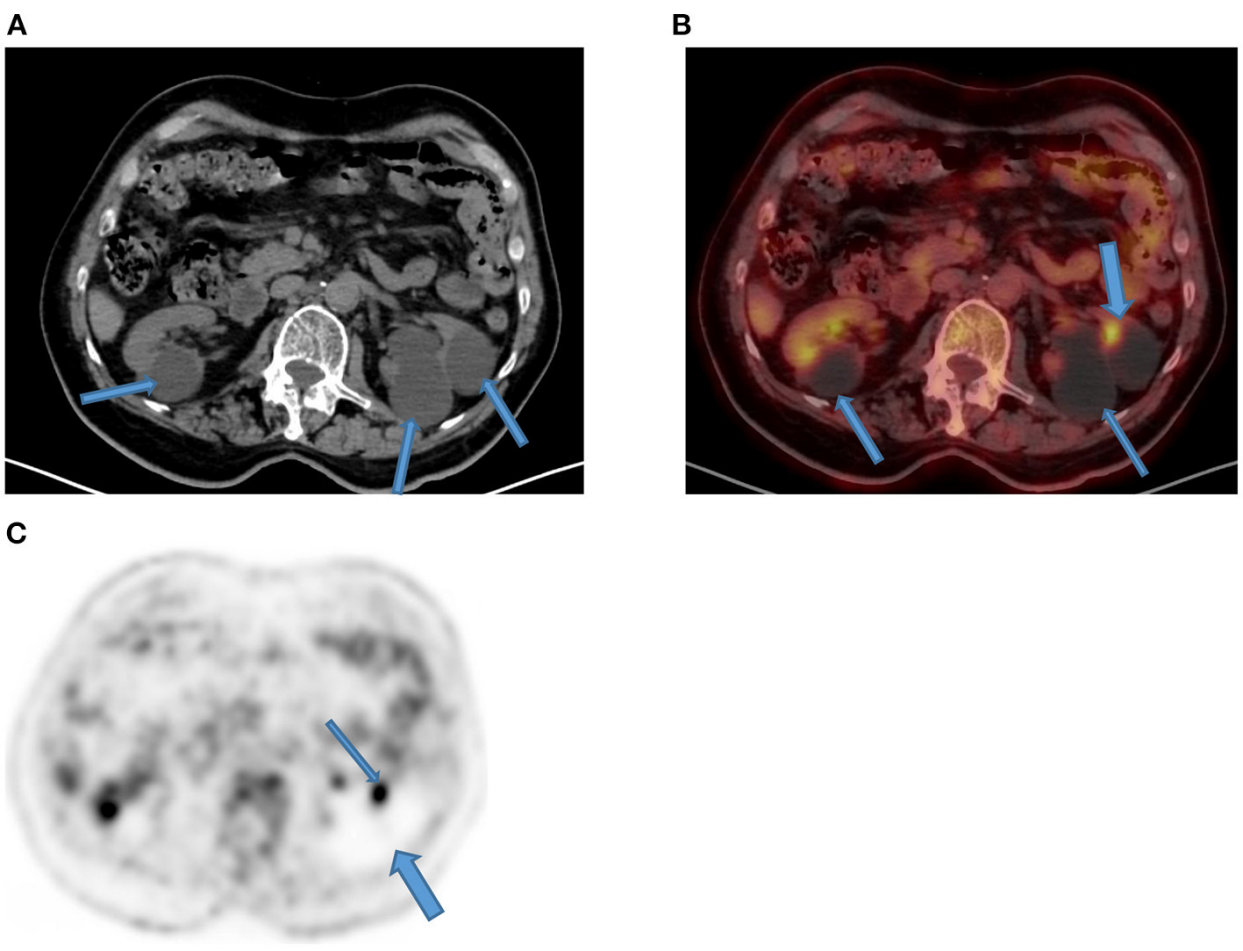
Focal kidney uptake. Axial CT (A) component of the PET/CT showed multiple bilateral Bosniak type 1 renal cysts that appear hypodense (arrows) as compared to thin normal renal tissue. Their magnitude in left kidney (thin arrows) showed compression and thinning of renal cortex with a focal FDG uptake (thick arrow) on corresponding combined PET/CT (B) that may mimic focal disease. Corresponding axial PET (C) demonstrates photon deficient areas due to renal cysts (thick arrow) with adjacent focal urinary uptake (thin arrow).
